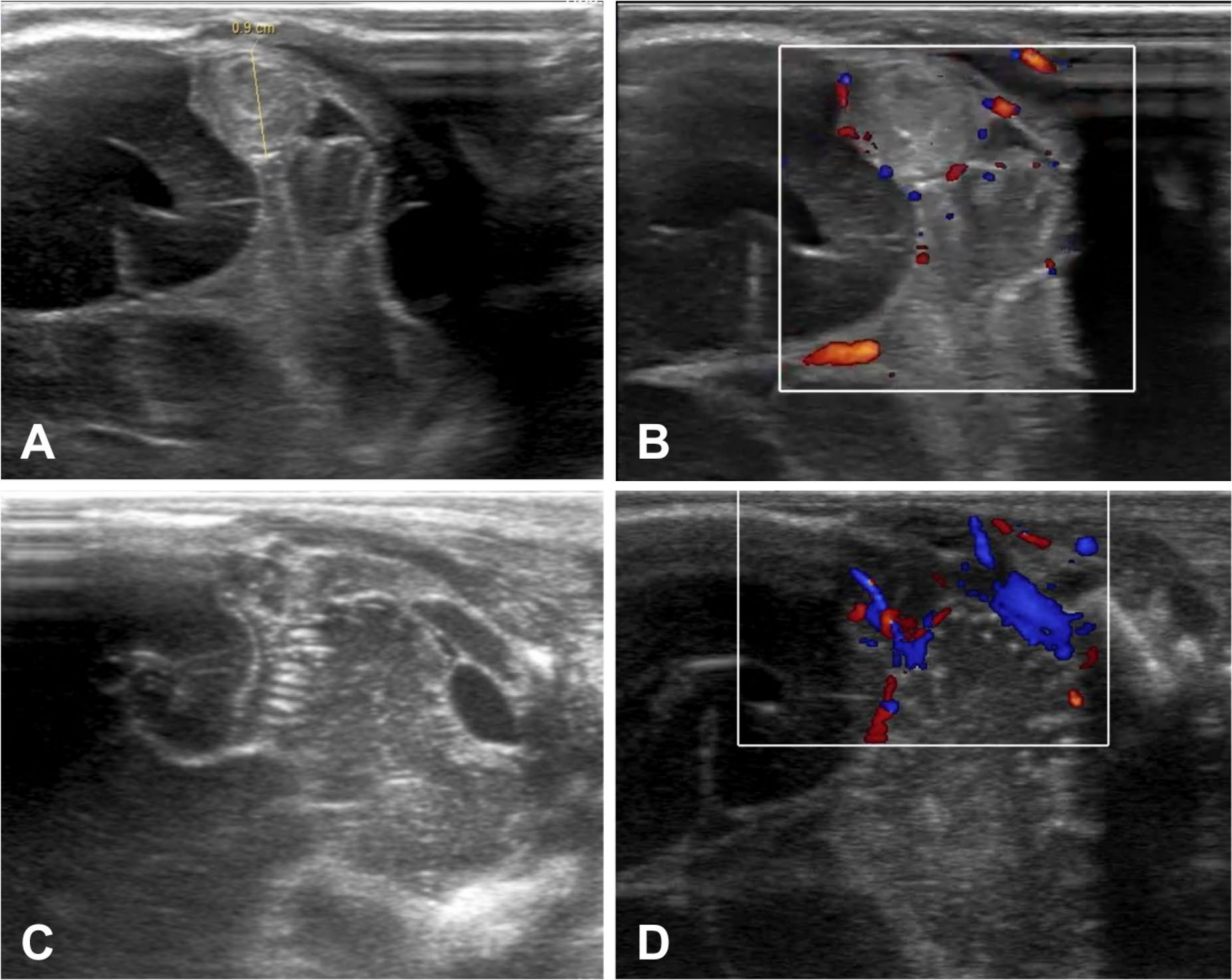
Cranial ultrasound imaging (diagnosing). A, B Ultrasound images taken during exam on the 16th day of life on right side (the day of CSVT diagnosis). C, D Ultrasound images taken during exam on the 16th day of life on left side
